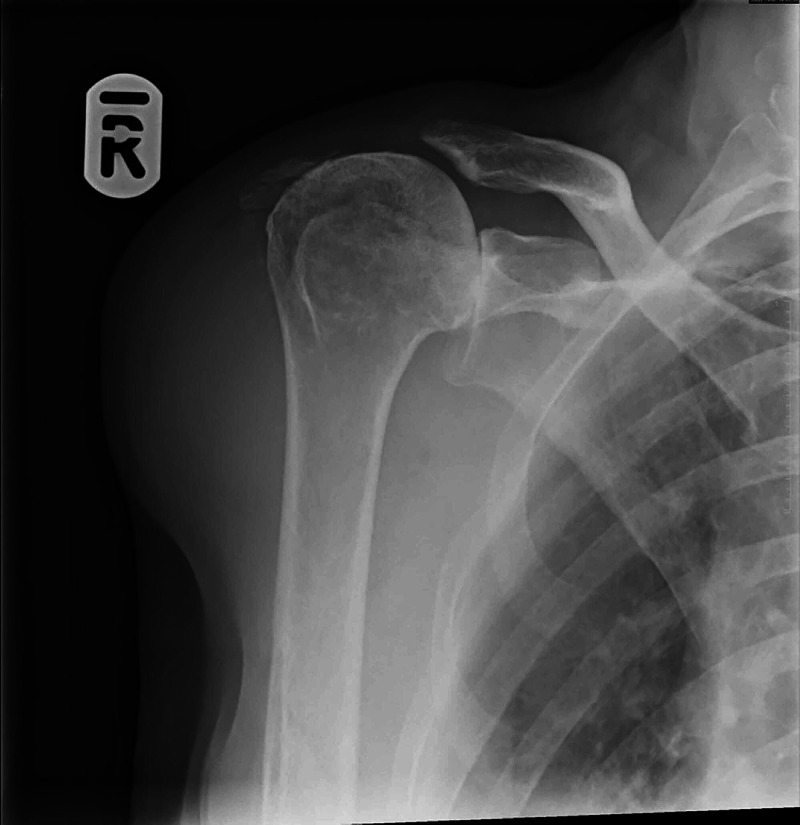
Radiograph of the right shoulder demonstrating superior migration of the humeral head and erosion of the acromion process with destruction of the acromioclavicular joint
